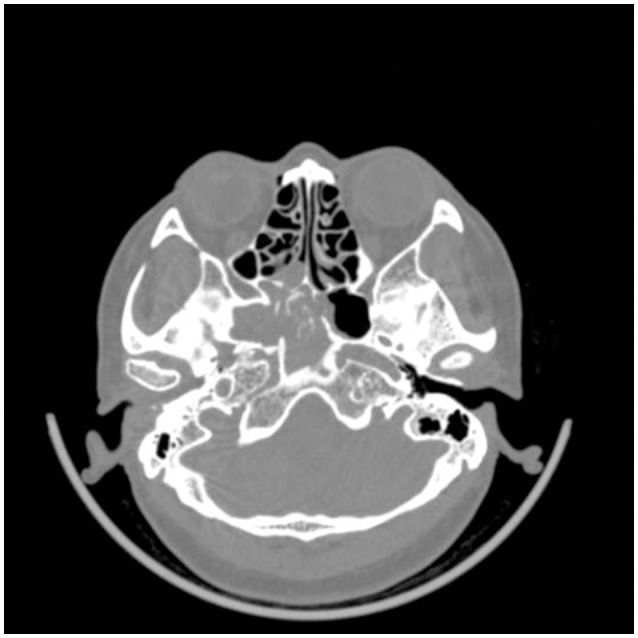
CT image of paranasal sinus. Irregular soft tissue intensity was observed in right sphenoid sinus with high density of point stripe calcification and lesion on the sinus wall.
